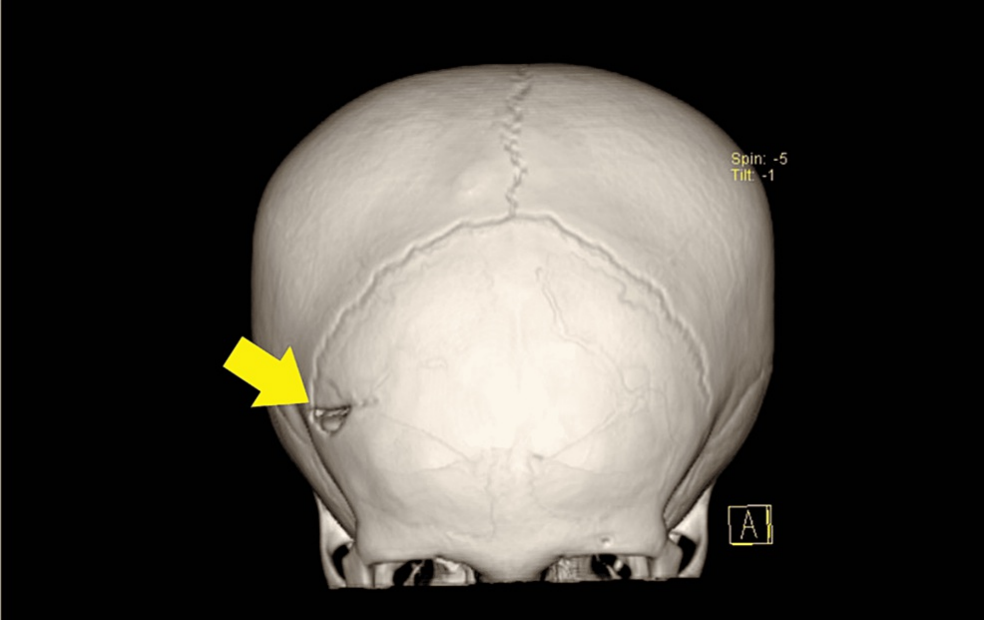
The yellow arrow shows the semilunar-shaped skull fracture at the right frontal bone in the three-dimensional-reconstructed computed tomography of the brain.
